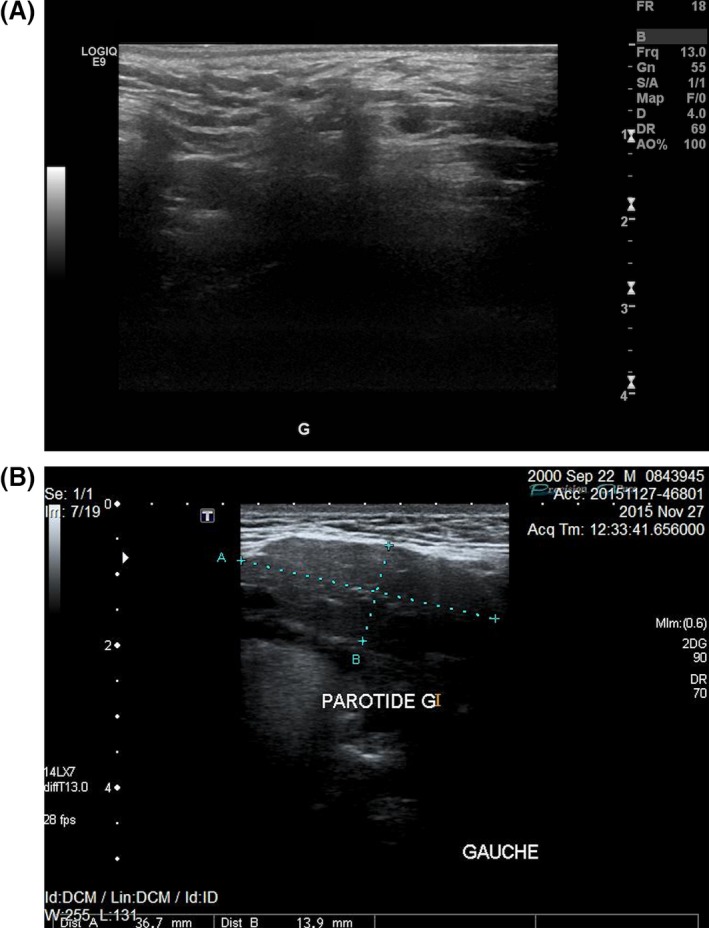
Ultrasonography demonstrating the complete absence of the left parotid gland in the presented case (A) compared to the ultrasonography of a normal parotid gland in a healthy 16‐year‐old male (B).
